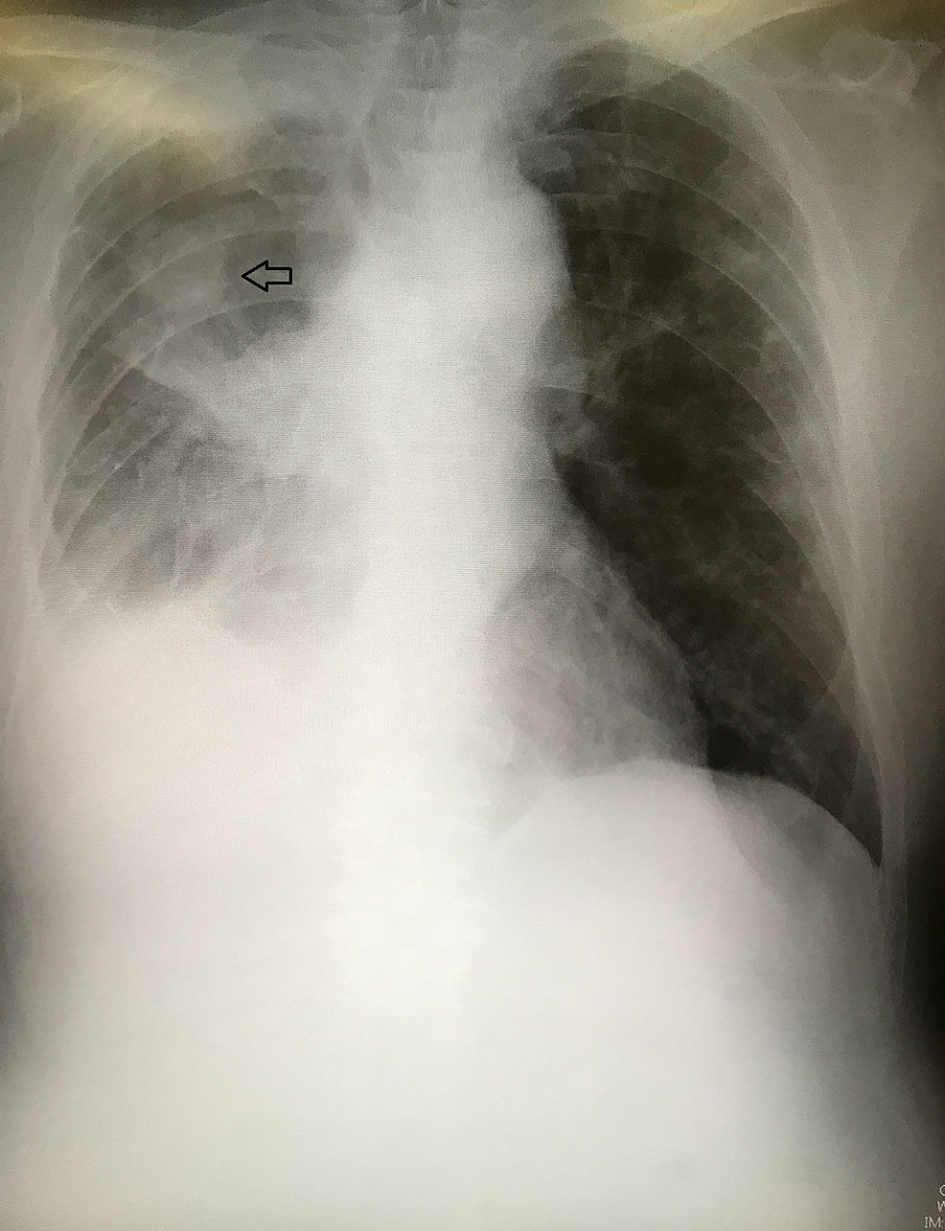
CXR showing the right lung adenocarcinoma and pleural effusion due to dissemination CXR: chest X-ray
